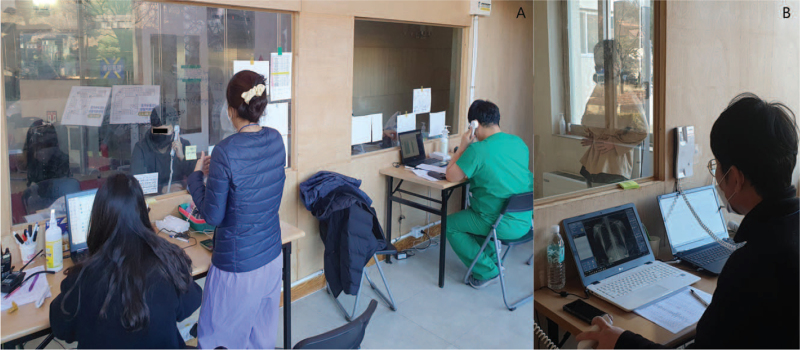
Noncontact medical room for identifying inpatient patients and performing x-ray, (A) Nurses and a doctor perform to consult and fill out medical card, (B) radiographer takes the chest x-ray.
